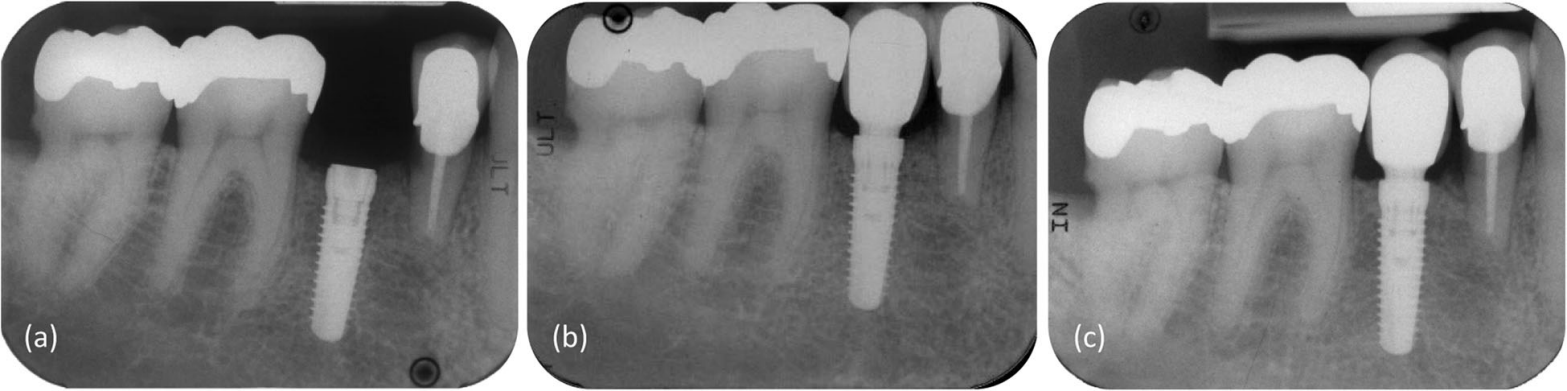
Standardized peri-apical radiographs representing the bone level changes at the implant level: immediately post insertion (a), at loading (abutment/crown placement) (b), and at 5-year post-loading (c)
